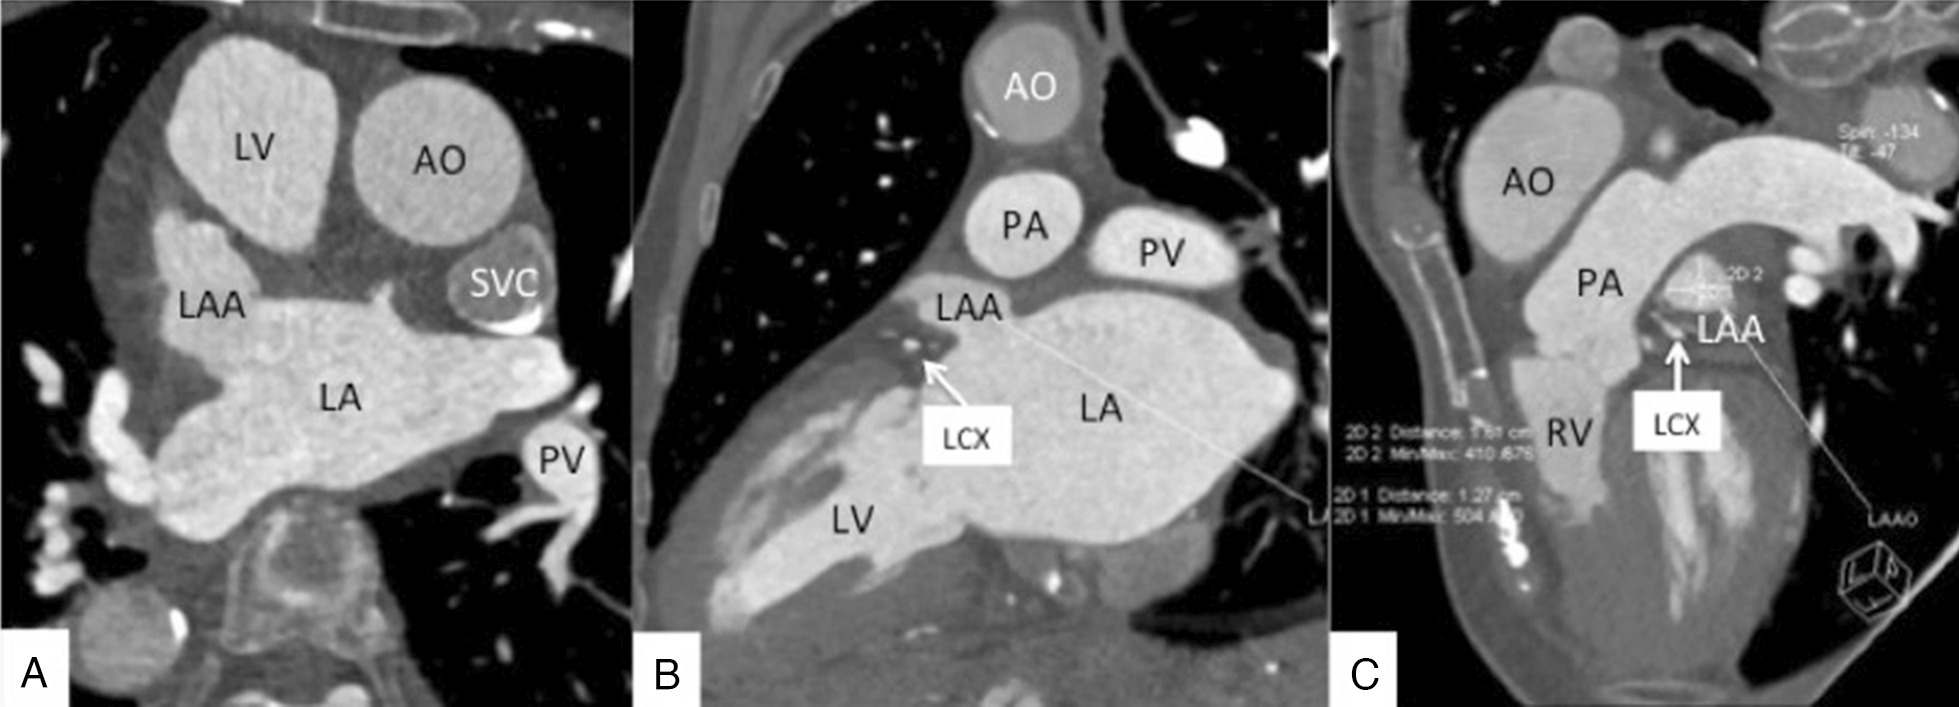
Computed tomographic angiography of the LA and LAA showing mirror dextrocardia. The LA and LAA had no filling defect in early- and late-phase images (A, B). Reconstruction images showing that the LAA ostial was 16 mm; the depth of the LAA was 19 mm (C). *AO* aorta, *LA* left atrium, *LAA* left atrial appendage, *LV* left ventricular, *PA* pulmonary artery, *PV* pulmonary vein, *LCX* left circumflex artery, *SVC* superior vena cava
